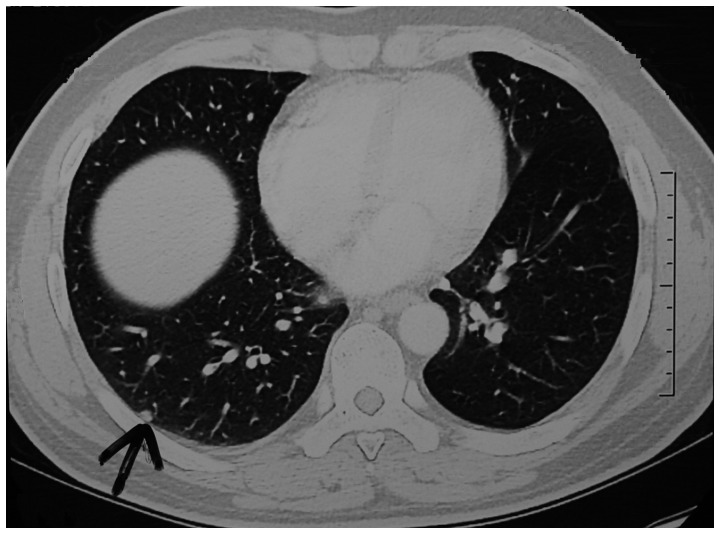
Case one. Chest computed tomography scan showing several newly developed nodules, the largest being 7 mm in diameter, at the last follow-up examination 33 months after the first surgery.
